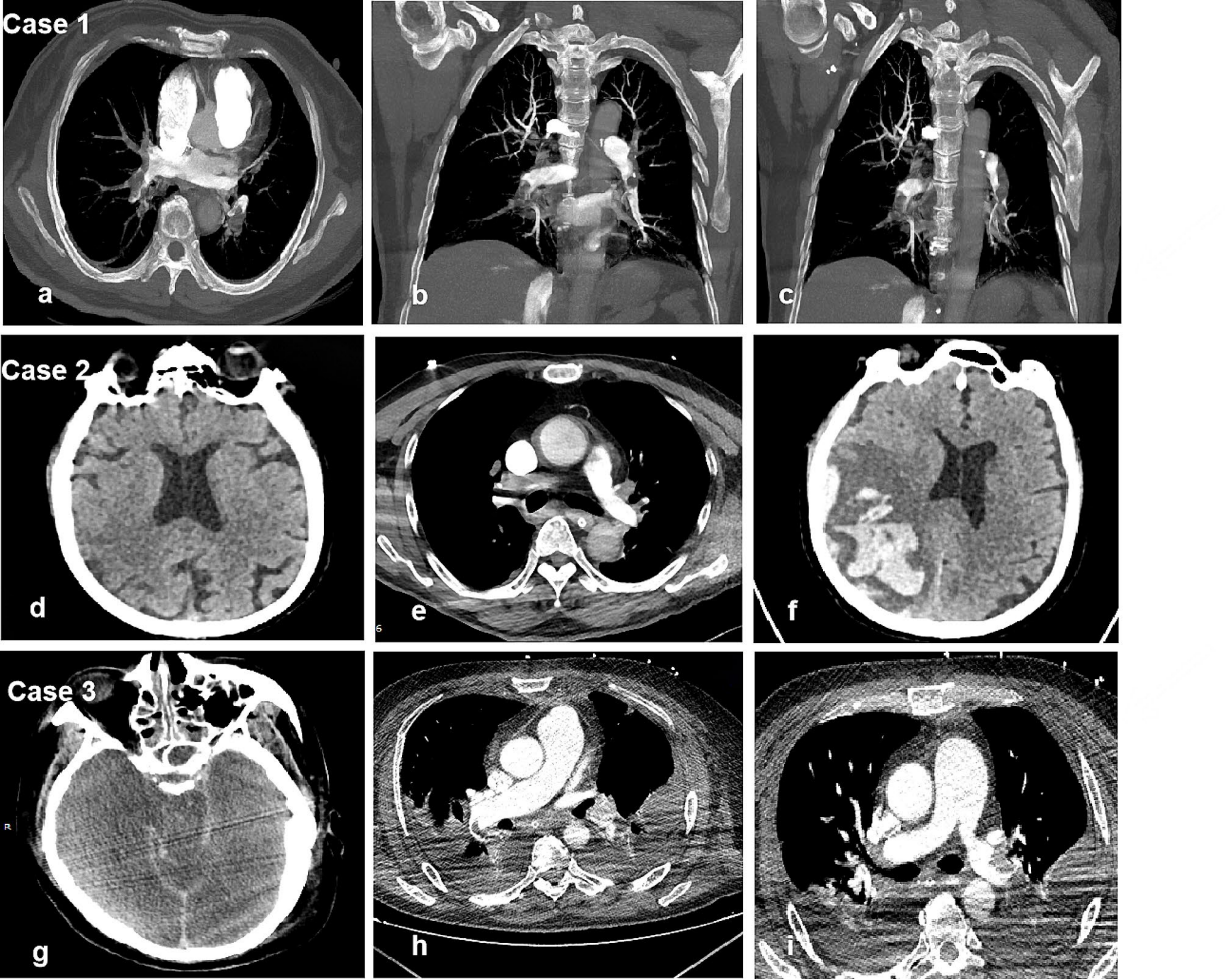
Images of 3 cases. Case 1 (a-c): Computed tomography pulmonary angiogram (CTPA) showed a bilaterally massive pulmonary embolism. Case 2: d, brain CT before thrombolysis was normal. e, CTPA revealed a massive pulmonary embolism. f, brain CT after thrombolysis showed hemorrhagic transformation following cerebral infarction. Case 3 : g, the brain CT revealed subarachnoid haemorrhage. h-i, CTPA showed massive left pulmonary artery embolism.
